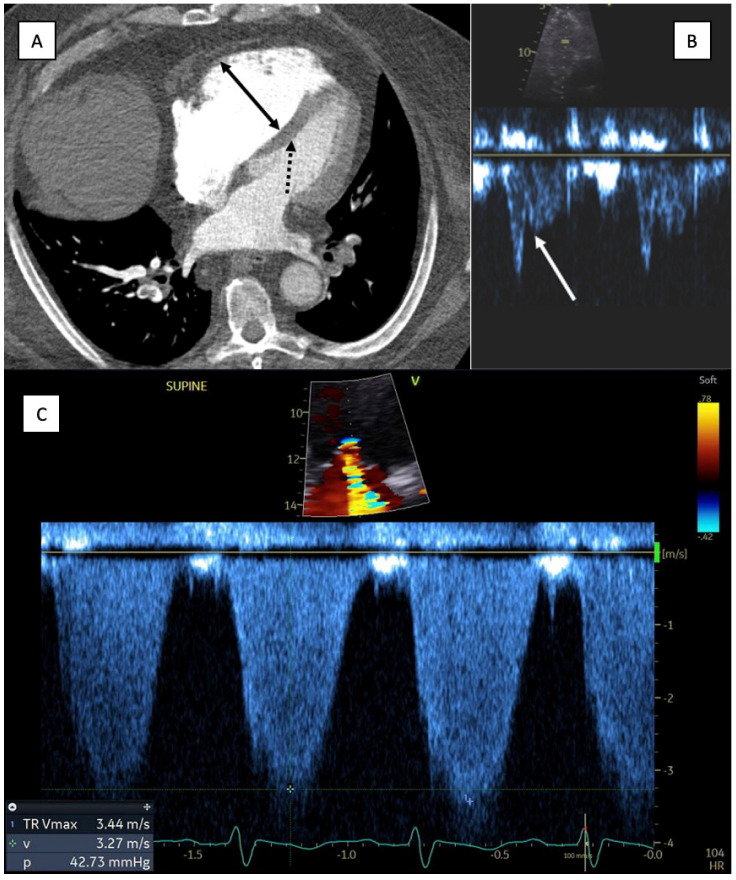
Computed tomography angiography findings consistent with right ventricular dysfunction [32]. (A) CTA chest: Axial image at the level of the mitral valve. Solid arrow demonstrating enlargement of the right ventricle compared to the left ventricular cavity. Dashed arrow highlights the deviation of the interventricular septum towards the left ventricle. (B) Transthoracic ECHO (same patient): shortened pulmonary ejection acceleration time (AcT) with a “notched” midsystolic velocity deceleration in the RV outflow track (white arrow). (C) Transthoracic ECHO (same patient): demonstrating tricuspid regurgitation (TR) peak systolic gradient (TRPG) of less than 60 mm Hg (42.7 mm Hg), consistent with the proposed 60/60 sign.
